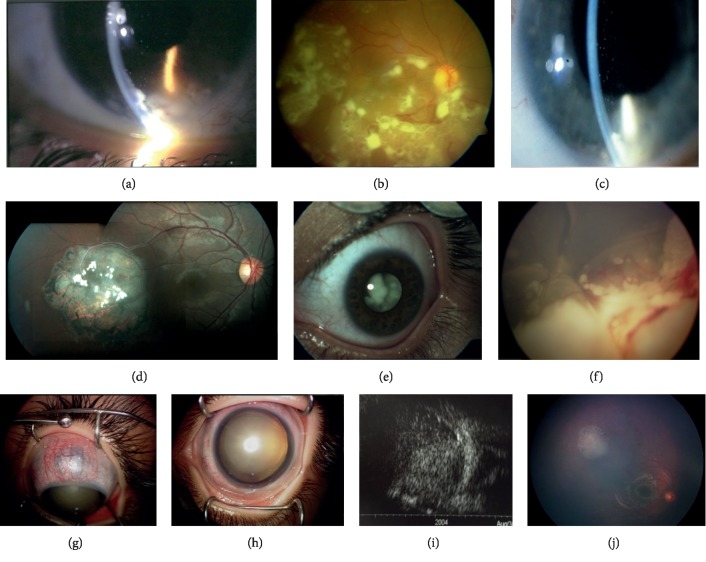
(a) Case #1. Anterior segment photo of the right eye in a 12-year-old-male patient showing multiple, round, whitish cystic lesions (pseudocysts) in the anterior chamber. (b) Fundus photo of the same patient demonstrating similar pseudocyts over the inferior half of the retinal surface. (c) Case #7. Anterior segment photo of the left eye in a 7-year-old female patient with nonconfluent pseudocysts in the anterior chamber. (d) Case #3. A 14-year-old male patient presented with a temporally located spontaneously regressed retinoblastoma in the right eye. (e) Anterior segment photo of the same patient demonstrating group E, endophytic retinoblastoma extending just behind the lens in the left eye. (f) Case #13. Fundus photo of the left eye in a 9-year-old female patient showing mixed endophytic-exophytic retinoblastoma with vitreous seeds in the inferior half of the fundus and total retinal detachment. (g–i) Case #14. A 9-year-old male patient was referred with a presumed diagnosis of Coats' disease in the left eye. Anterior segment photo showing rare coexistence of anterior staphyloma due to neovascular glaucoma (g), cataract, and ectropion uvea (h). Ultrasonography of the left eye demonstrating solid tumor with calcifications filling the vitreous cavity (i). (j) Fundus photo of the same patient showing a spontaneously regressed retinoblastoma located superotemporally in the fellow (right) eye.
